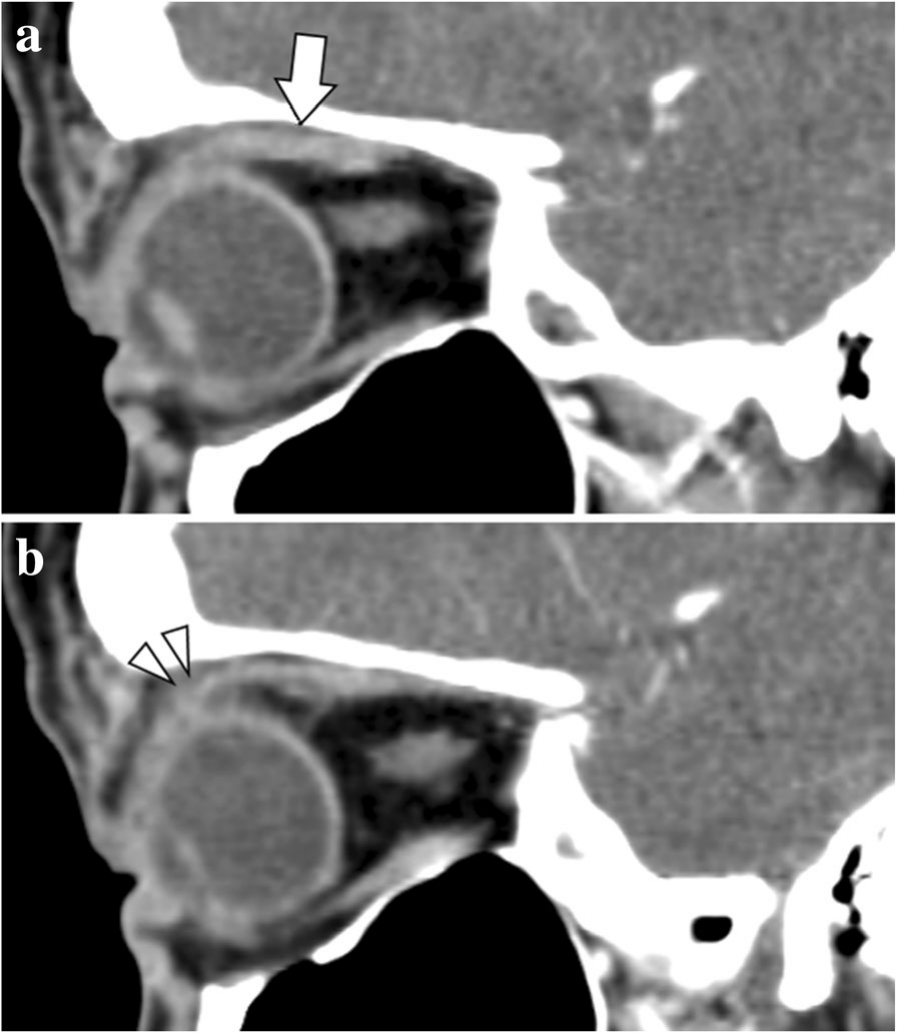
CT sagittal view scan at the first visit. a Suspicious enlargement of the right SR muscle was observed (arrow: the right SR muscle). b The infiltration around the SR muscle insertion were observed to be increased. The insertion of the SR muscle was not clearly shown in the CT scan (arrow head: ambiguous insertion of the SR muscle)
